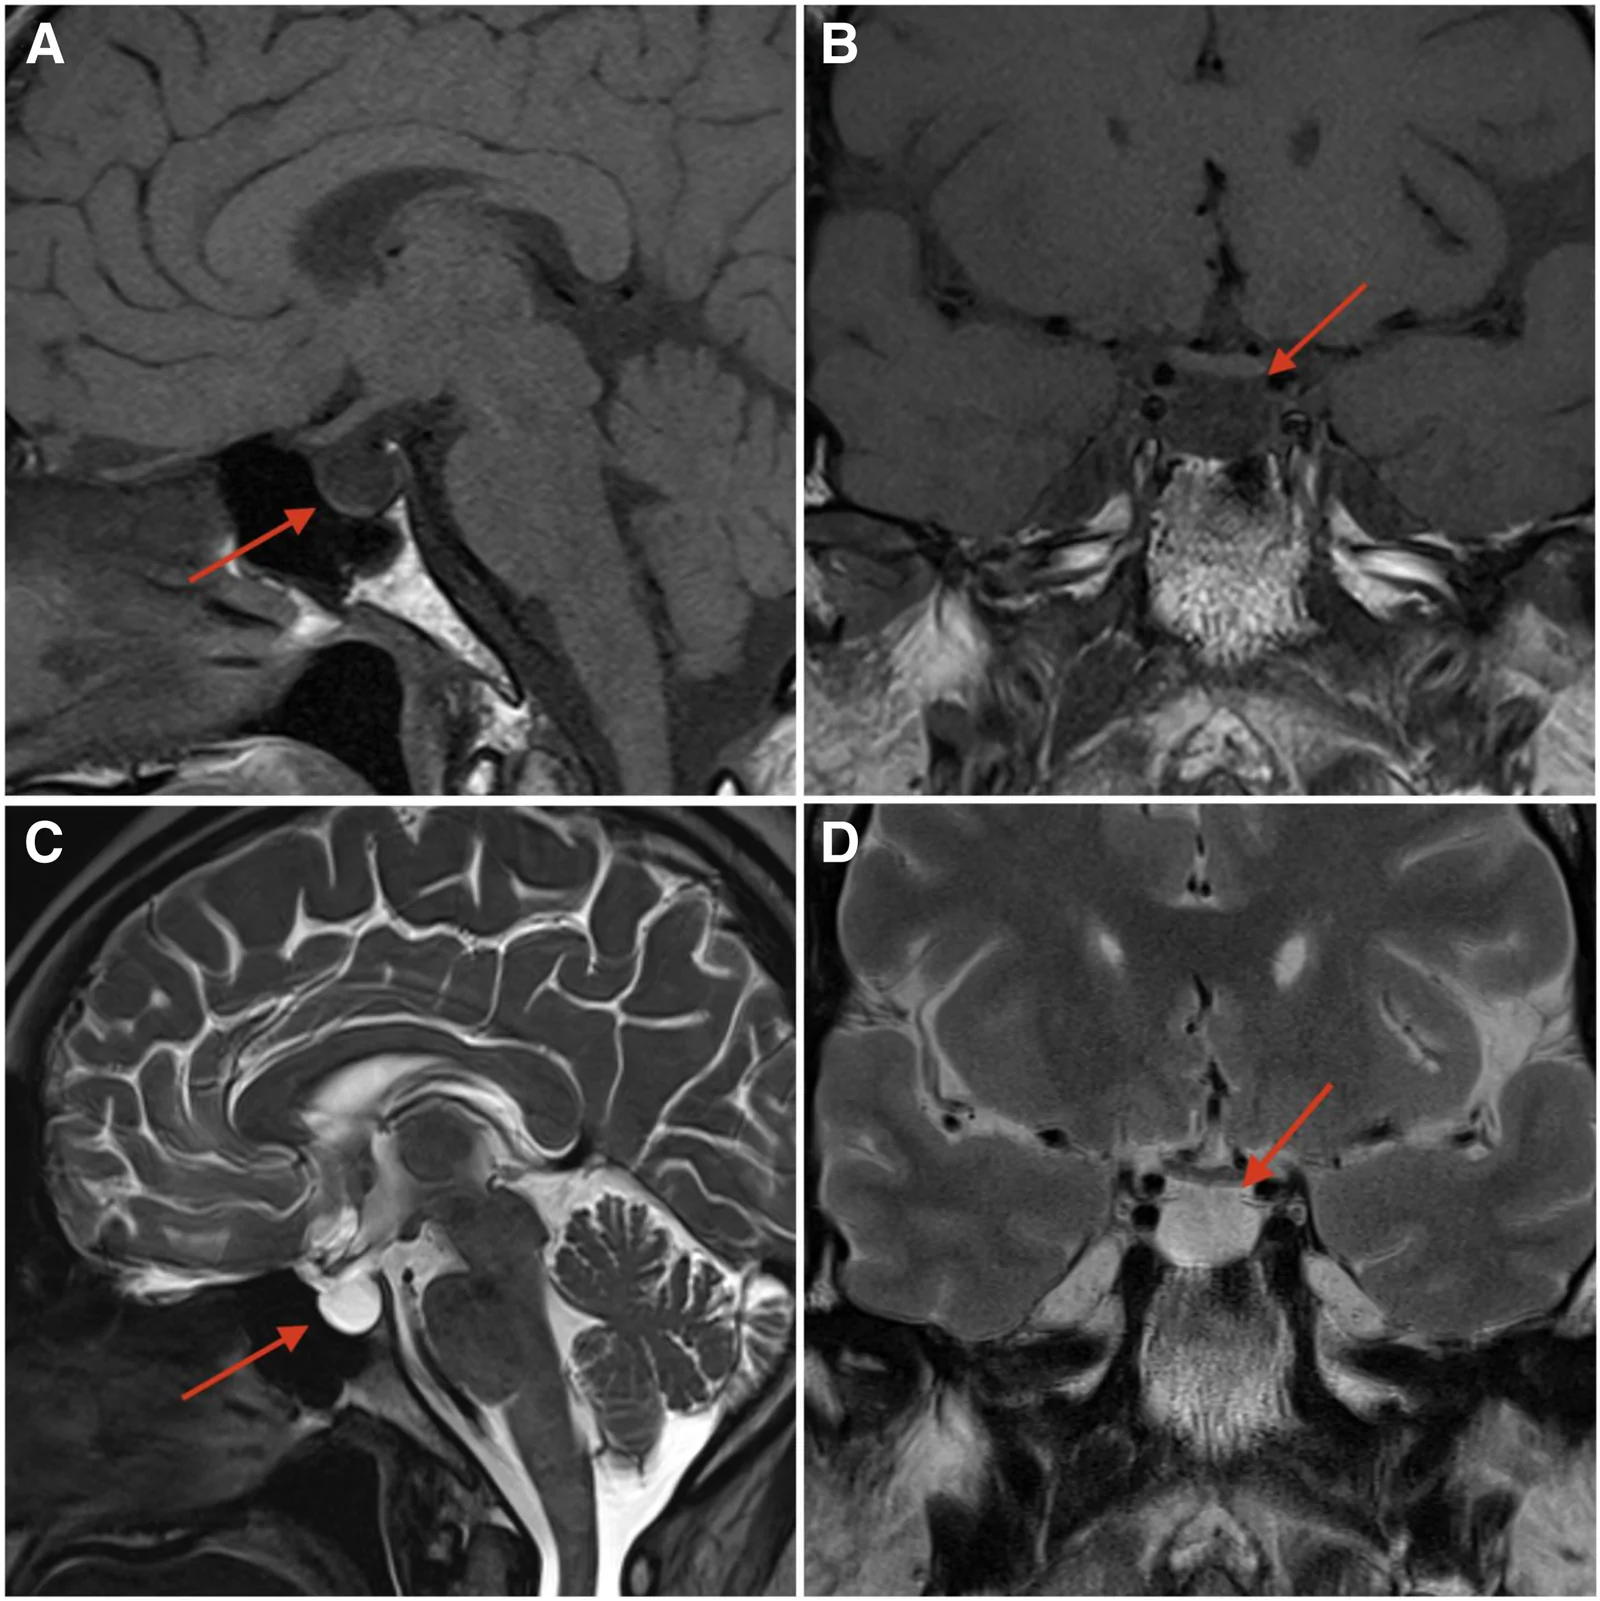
Pituitary magnetic resonance imaging showing an empty sella with mild leftward deviation of the pituitary stalk. T1-weighted sagittal (A) and coronal (B) sections. T2-weighted sagittal (C) and coronal (D) sections.
